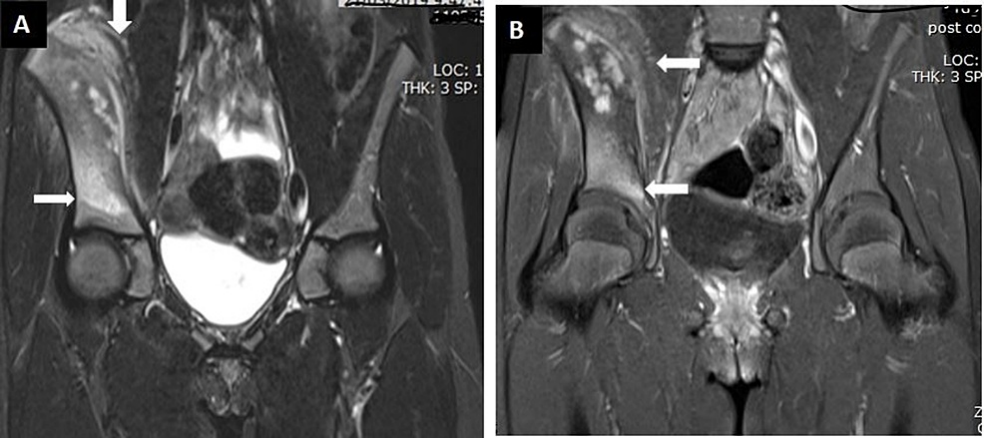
MRI of the pelvis (coronal sections). A: STIR sequence showing abnormal signal enhancement in the right ilium extending to the acetabular roof (horizontal white arrow). It also displays abnormal signal enhancement in the right iliacus muscle (vertical white arrow). B: T1 post-contrast sequence displaying enhancement in the affected areas in the right ilium (white arrow). STIR, short tau inversion recovery
